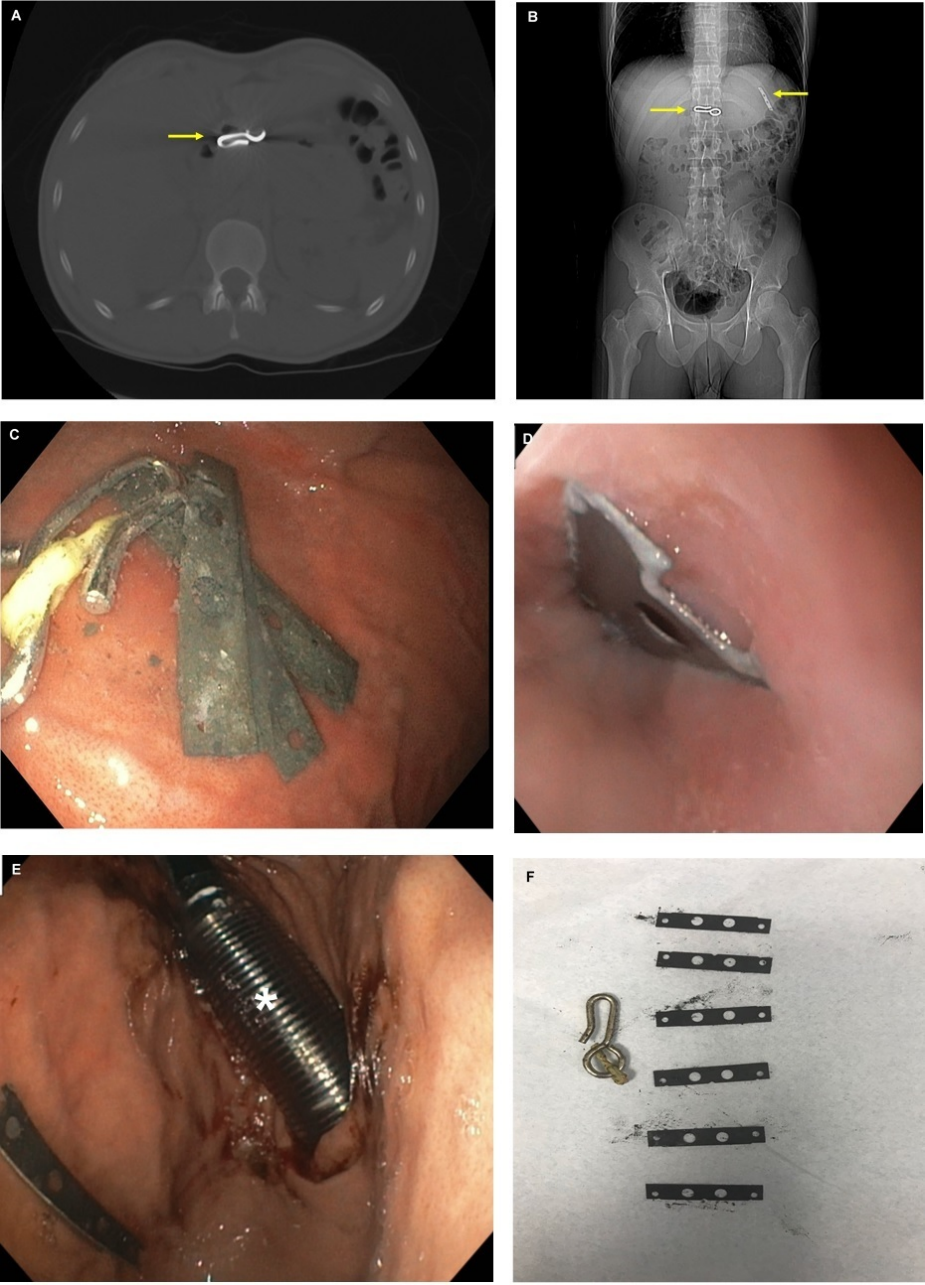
A – Coronal computed tomography scan of a curtain hook (arrow). B – Axial computed tomography scan showing a curtain hook and a single razor blade (arrows). C – Endoscopic visualization of razor blades and a curtain hook in the stomach. D – Razor blade seen at the gastroesophageal junction. E – Overtube (asterisk) was used to protect the mucosal wall during foreign body removal. F – Six razor blades and a curtain hook were sequentially removed from the body of the stomach.
